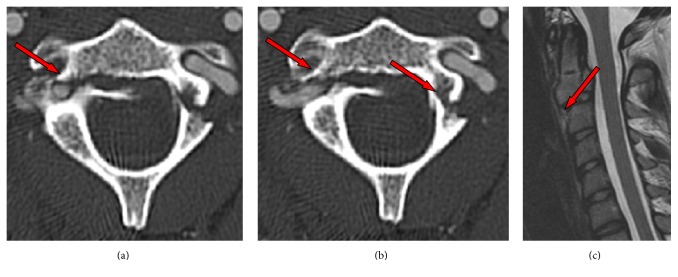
((a) and (b)) Primary CT with contrasted dislocation of the artery in the fracture gap; (c) sagittal MRI arrows point to a small hematoma under the anterior longitudinal ligament.
